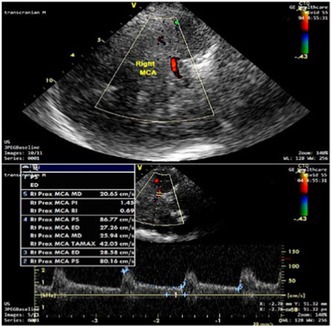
FIGURE 1 Transcranial Doppler ultrasound recording of the middle right cerebral artery (MCA). The spectrum shows peak systolic velocity (PSV), end‐diastolic velocity (EDV), and mean blood flow velocity (MBFV) used for the calculation of the pulsatility index (PI) and resistivity index (RI).
